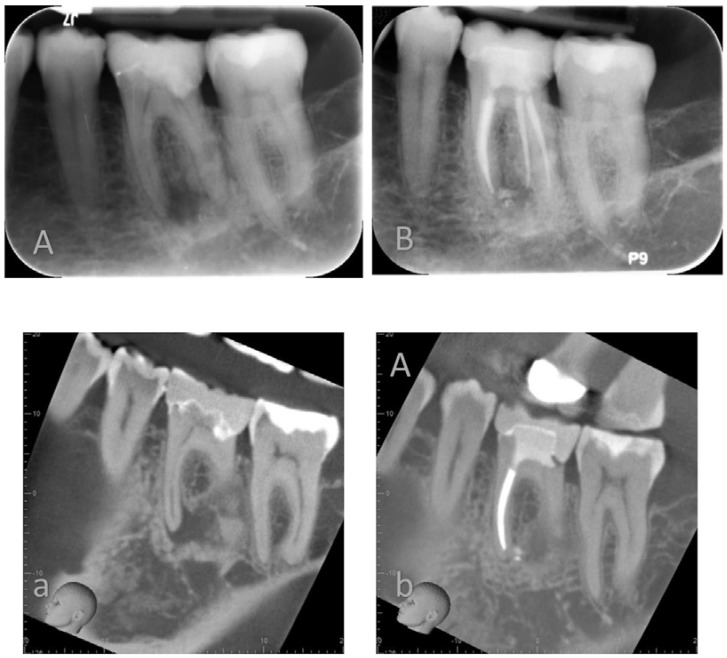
Lower left mandibular first molar root filled using a warm vertical condensation technique and AH plus root canal sealer. Preoperative Periapical X-ray (A) and Cone Beam Computer Tomography (a) and recall PA X-ray (B) and CBCT (b) at 12 months.
